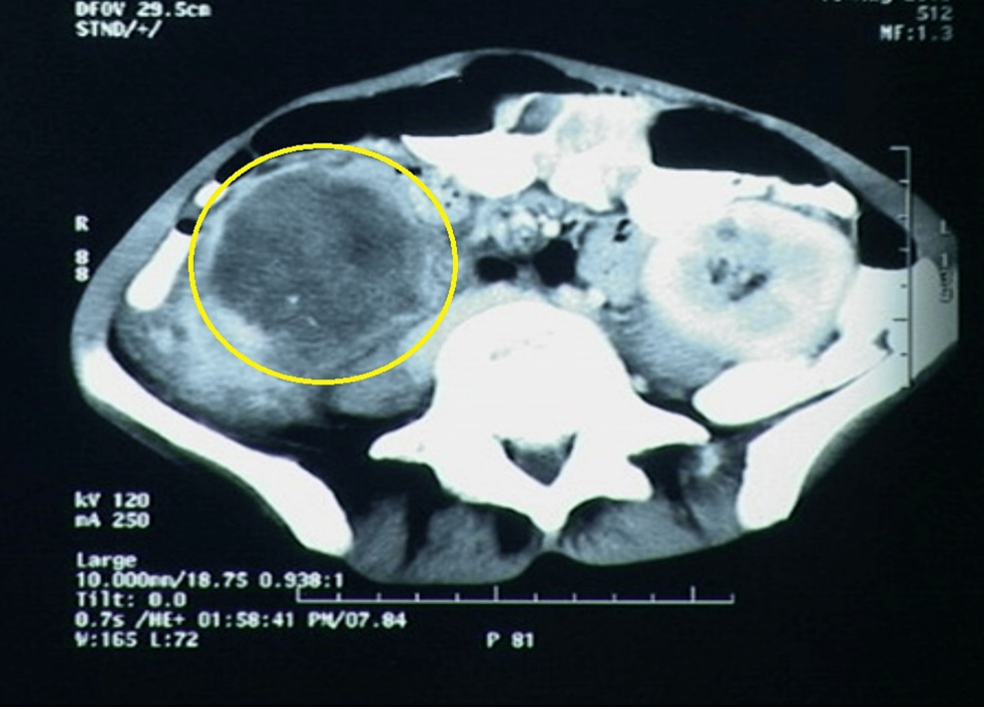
Lesion as observed on the axial section of the CECT scan abdomen showing an irregular hypodense mass lesion (yellow circle) measuring 87 x 67 mm in the axial plane in the mid and lower pole of the right kidney having internal calcific foci and few renal calculi occupying the renal pelvis CECT: Contrast-enhanced computed tomography
